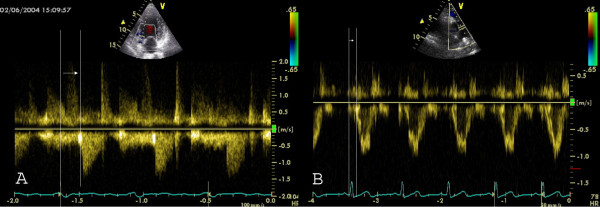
Measurement of interventricular mechanical (IMD) delay by PW Doppler: A) PW Doppler in aortic outflow tract: Measurement from onset of QRS to the onset of PW curve in the aortic outflow tract. This time is also called the aortic pre-ejection time and is a marker for intra(left)ventricular asynchrony. B) PW Doppler in pulmonary outflow tract: Measurement from onset of QRS to the onset of PW curve in the pulmonary outflow tract. The IMD is the difference between the time of a) and b).
